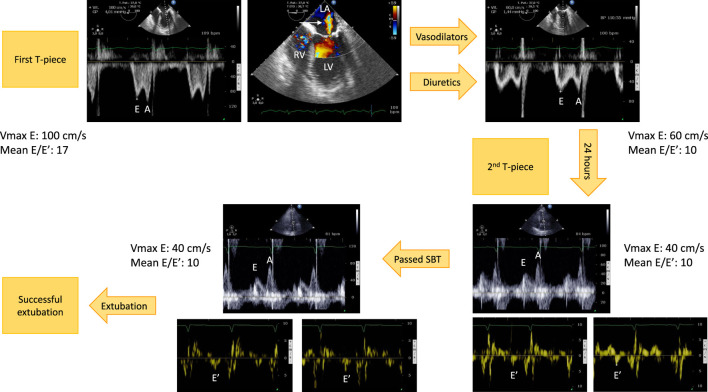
Example of hemodynamic monitoring using echocardiography in a patient with left ventricular systolic dysfunction secondary to an ischemic heart disease who developed a weaning-induced pulmonary edema during the first T-piece trial which induced high blood pressure. Immediately after having reconnected the patient to the ventilator, a transesophageal echocardiography was performed. Mitral Doppler depicted elevated E wave velocity and mean E/E′ ratio, consistent with increased left ventricular filling pressure (upper left panel). There was only a mild functional mitral regurgitation (upper middle panel, arrow). Treatment associated short half-life sedative, ACE inhibitors and diuretics. With normalization of blood pressure and fluid removal, the mitral Doppler profile remained unchanged (E/A ratio ≈1), but velocities significantly decreased as well as mean E/E′ ratio (upper right panel). This allowed to perform a second T-piece trial 24 h later. Immediately before the procedure, mitral E wave velocity remained low, E/A ratio was <1, and mean E/E′ remained stable, denoting the absence of new rise in left ventricular filling pressure (3 lower right panels). The second spontaneous breathing trial was clinically successful. Echocardiography at the end of the 30-min T-piece trial depicted similar mitral Doppler velocity values, hence left ventricular filling pressure (3 lower left panels). The patient was then extubated successfully without any change of ongoing treatment. Abbreviations: LA, left atrium; LV, left ventricle; RV, right ventricle.
